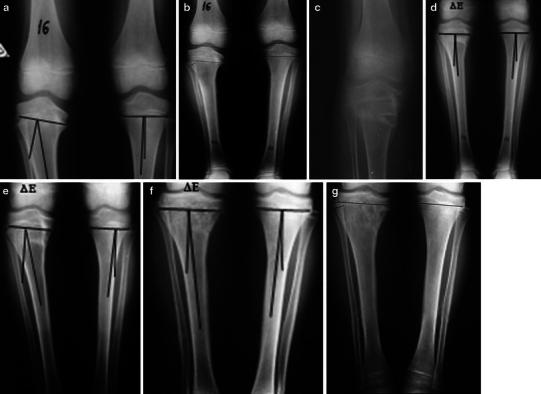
a Anteroposterior radiographs of a 13 year old boy with an S-H type V proximal physeal injury of the right tibia, 12 months post-injury. b Anteroposterior radiographs of the same patient 12 months post-injury, showing a tibial length discrepancy of 6 mm. c The patient underwent a correctional osteotomy. d Five months after the correctional osteotomy, without angular deformity. e Two years after the correctional osteotomy. Malalignment of the tibial axis is present. f Four years after the correctional osteotomy. Despite the partial remodeling, a residual valgus deformity of 2° can be observed. g Four years after the correctional osteotomy. No tibial or fibular length discrepancy is apparent
